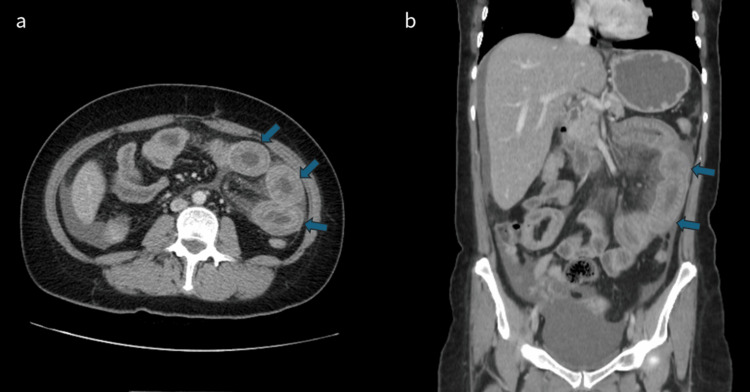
Second contrast-enhanced computed tomography (CT) scan of the abdomen and pelvis. In the axial (a) and coronal section (b), we can see circumferential wall thickening of the proximal jejunum (arrows) with submucosal edema and mucosal hyperenhancement.
